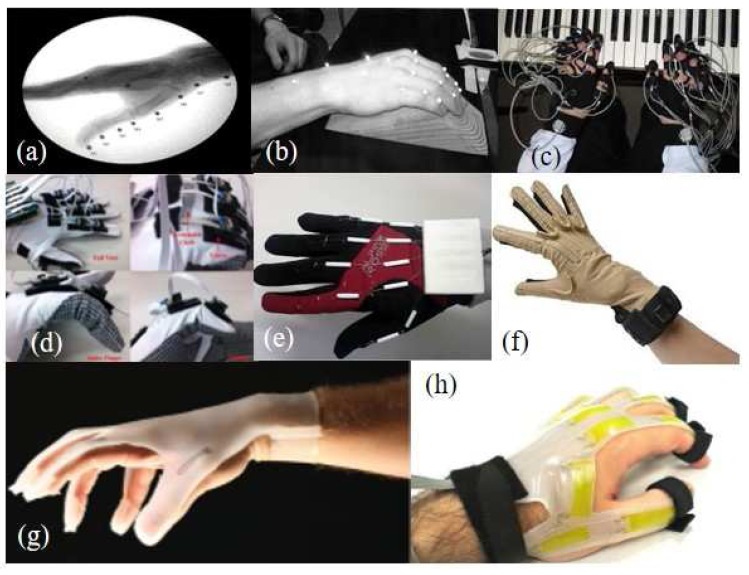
Examples of finger motion measurement systems: (a) X-ray based system [2]; (b) Infrared (IR) camera based system [3]; (c) Three dimensional position sensor system [4]; (d) Optical linear encoder based system [5]; (e) Potentiometer-based system [6]; (f) CyberGlove [7]; (g) A glove with embedded strain sensors [8]; (h) Wearable soft artificial skin [9].
